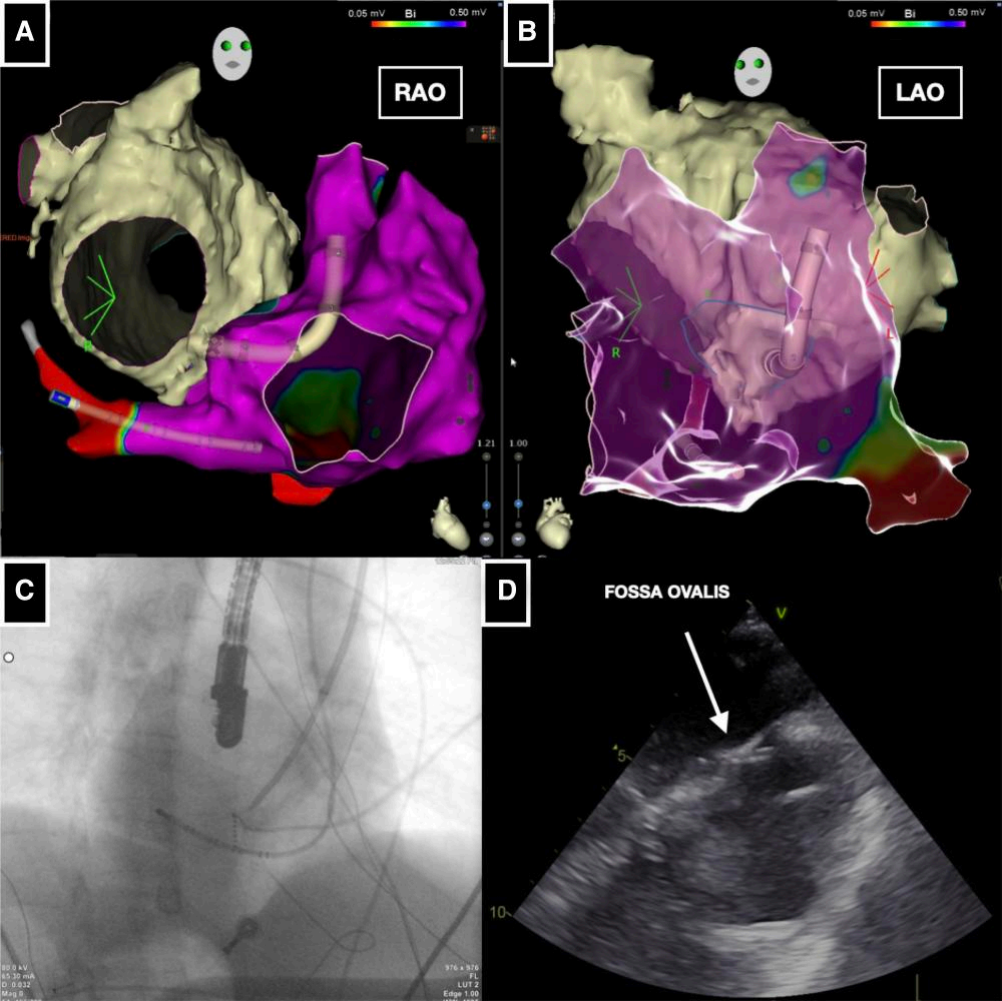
(*A* and *B*) Electroanatomical mapping [RAO projection mimicking LAO projection (*A*) and LAO projection mimicking RAO projection (*B*)] with cardiac magnetic resonance integration guiding the positioning of the VIZIGO sheath at the fossa ovalis. (*C* and *D*) Fluoroscopy RAO projection (*C*) and transoesophageal echocardiography (*D*) guiding the positioning of Fast-Cath long sheath at the fossa ovalis.
